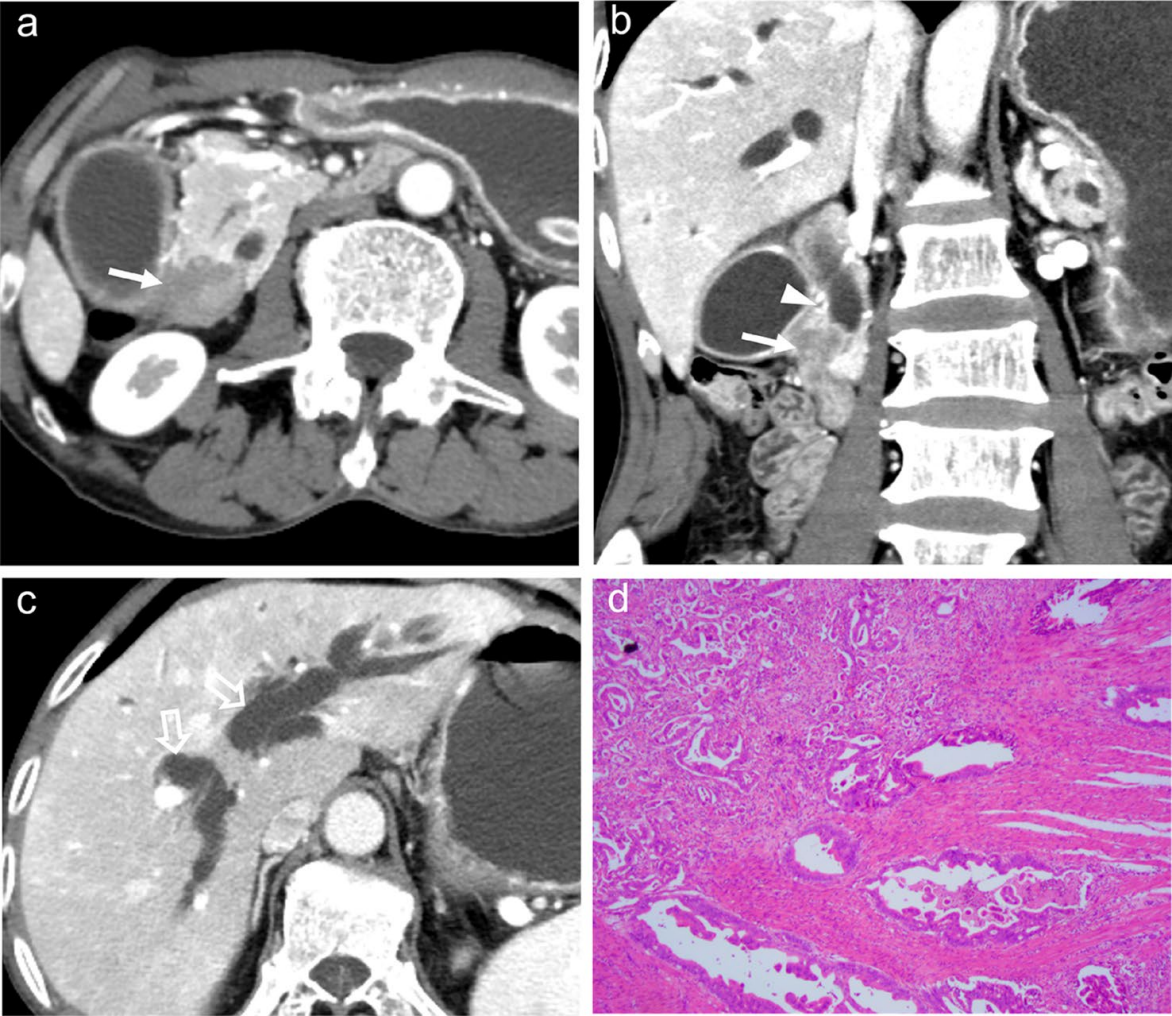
An example from a 65-year-old man who presented with jaundice. Axial (a, c) and coronal reconstructed images (b) of enhanced CT in portal venous phase show a periampullary mass with enhancement degree non-similar to that of the duodenal wall (arrows), with extrinsic growth pattern. The shape of distal CBD margin is irregular (arrowheads) accompanied by corresponding upstream bile duct dilatation (open arrow). The postoperative pathologic diagnosis of this patient was a pancreatobiliary-type periampullary adenocarcinoma (d)
